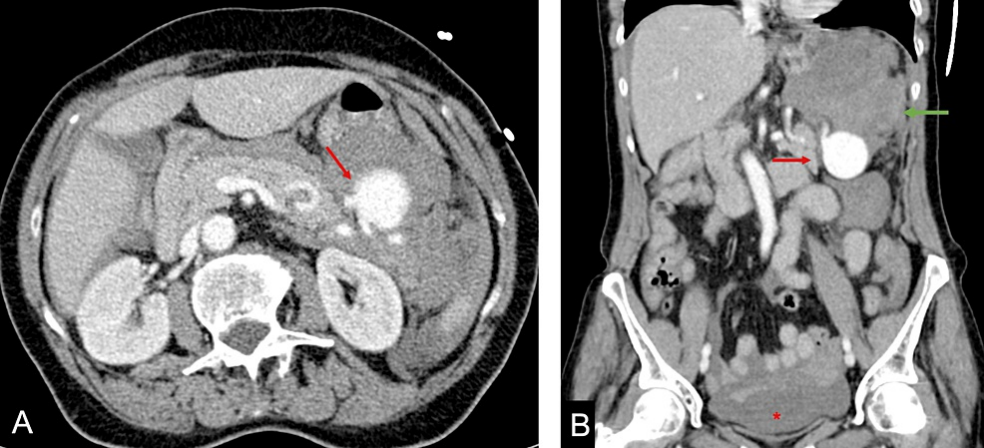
Contrast-enhanced CT axial (A) and coronal (B) reconstruction showing a saccular splenic artery aneurysm (red arrows), with adjacent hematoma (green arrow) and hemoperitoneum (asterisk).
